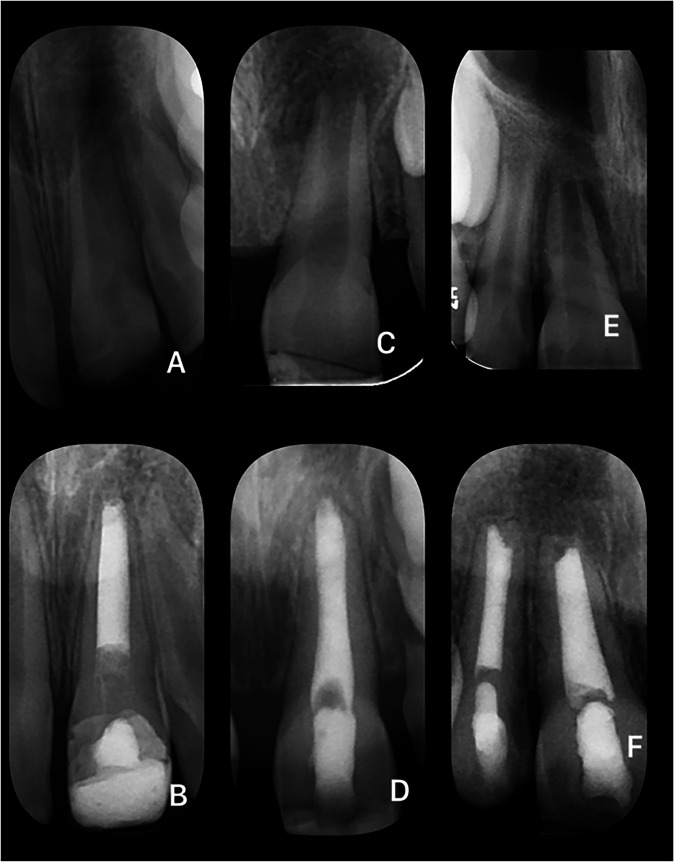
Pre- and post-operative radiographs of example cases with a “Favourable” outcome. A Maxillary central incisor in a patient between 5 and 10 years old with a history of enamel-dentine fracture (Pre-op). B The same tooth at 8 months post-treatment. C Maxillary central incisor in a patient between 5 and 10 years old with a history of avulsion (Pre-op). D The same tooth at 11 months post-treatment. E Maxillary central and lateral incisors in a patient between 5 and 10 years old with a history of avulsion and enamel-dentine fracture (Pre-op). F The same teeth at 7 and 18 months post-treatment.
